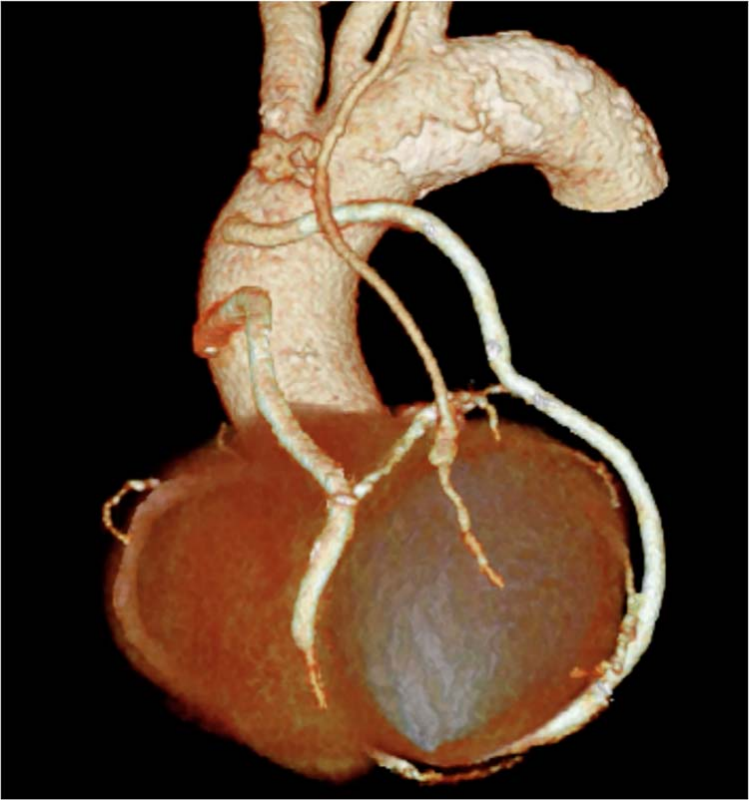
Postoperative 3D CT image showing the bypass graft design.
